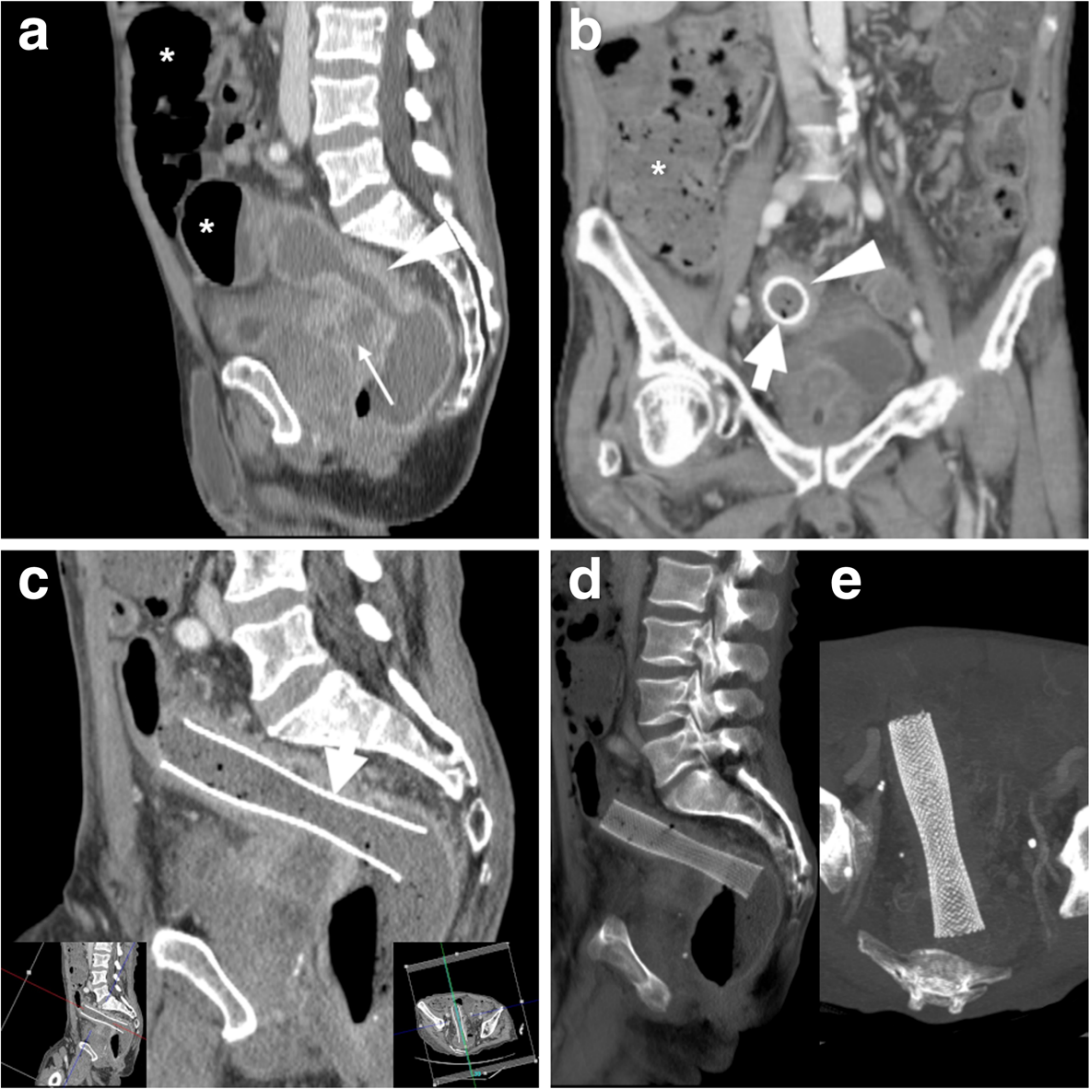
Palliation of unresectable CRC and dedicated CT reconstruction techniques. In an elderly male, sagittal contrast-enhanced CT (a) shows a 6-cm-long segment with enhancing, non-stratified increased mural thickness (arrowhead) at the rectosigmoid junction, causing luminal stricture and upstream large bowel obstruction (LBO*) complicated by fistulisation (thin arrow) to the prostate and urinary bladder. After endoscopic stenting, repeated CT (b, c) including focused oblique images (note obliquity in c insets) effectively depicted the correct position of the SEMS (thick arrows) at the site of CRC (arrowheads), filled with faecal fluid with partial persistence of upstream colonic dilatation (*). Additional thick-slab (d) and maximum-intensity projection (MIP) reconstructions depict the tubular shape and reticular “mesh” structure of the well-expanded SEMS (e)
